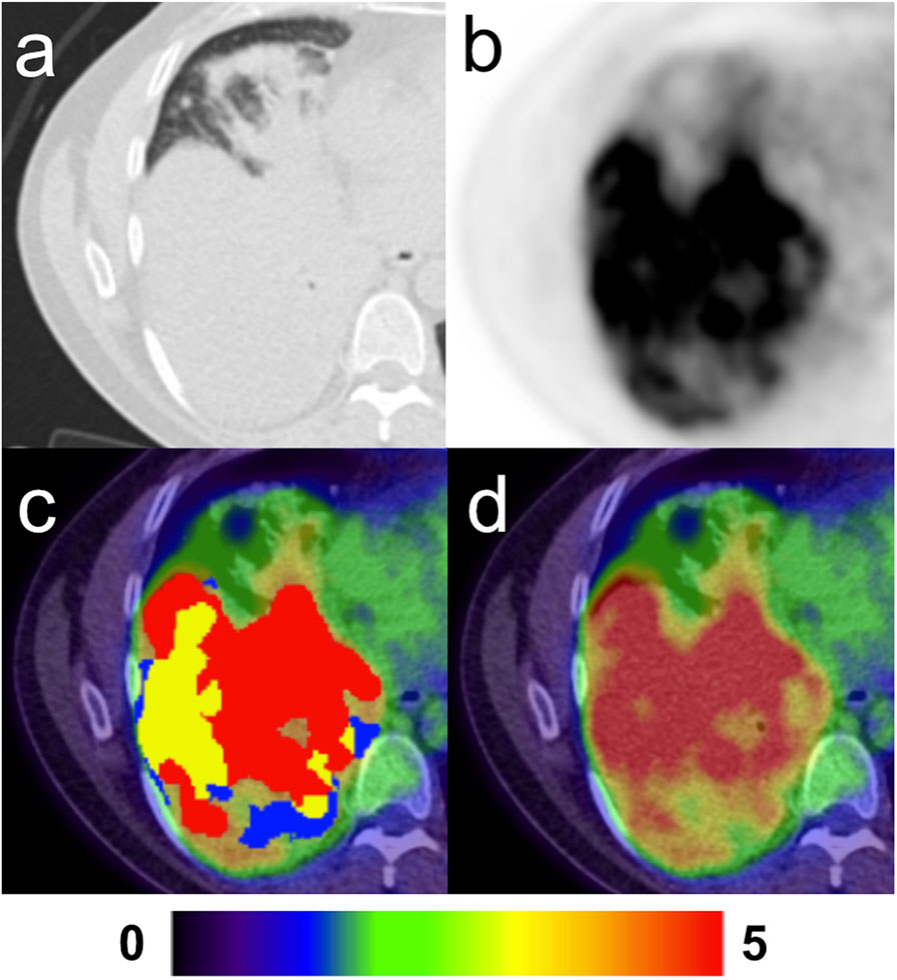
Large lesion with complex necrosis in the right lung. a Axial CT with lung window. b Axial PET. c Fused axial PET-CT with overlaying segmentations; manual only (red), AI-only (blue) and manual + AI (yellow). d Fused axial PET-CT
